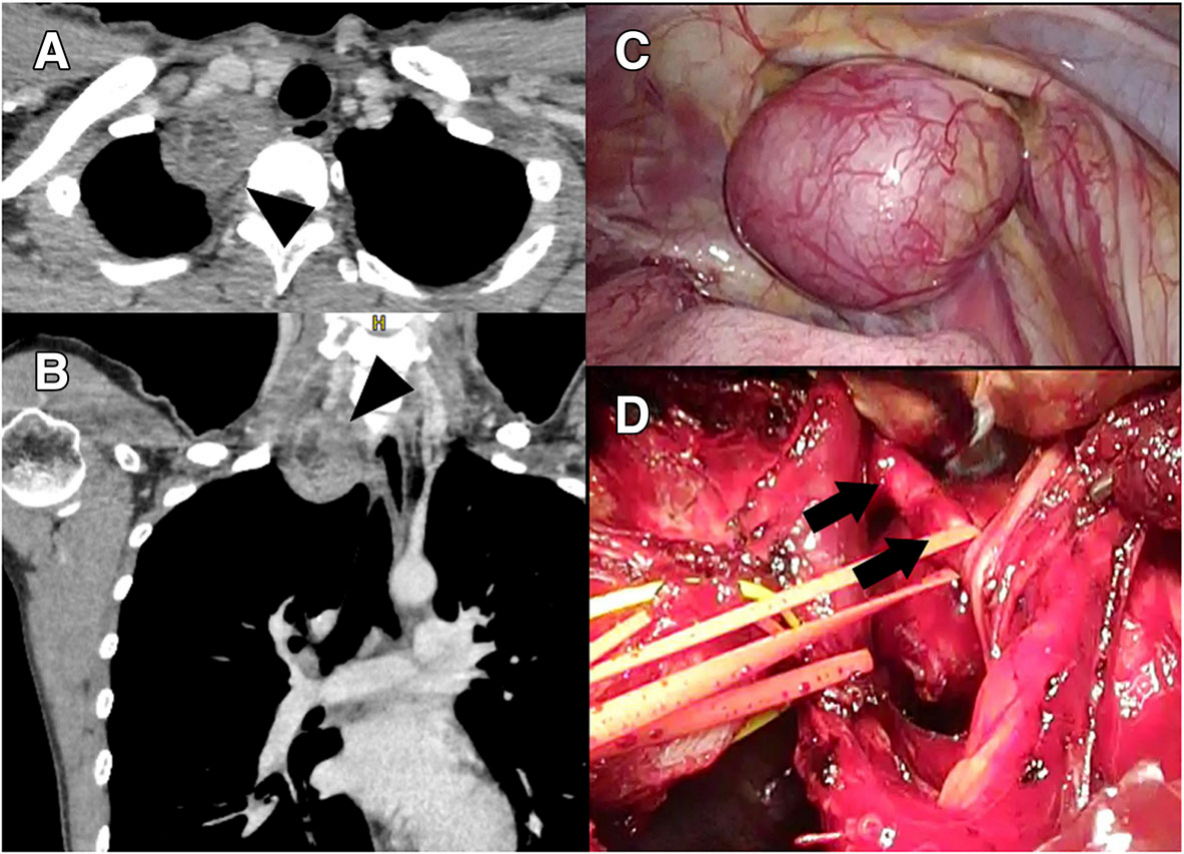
Axial (a) and coronal (b) views of the chest computed tomogram. The tumor (arrowhead) was in the thoracic outlet and adjacent to the right brachiocephalic artery and right subclavian vein. Intraoperative findings with video-assisted thoracoscopic surgery (VATS) (c) and the transmanubrial osteomuscular sparing approach (TMA) (d). The tumor was safely resected through the dual approach of VATS and TMA after encircling the right brachiocephalic artery (arrow)
